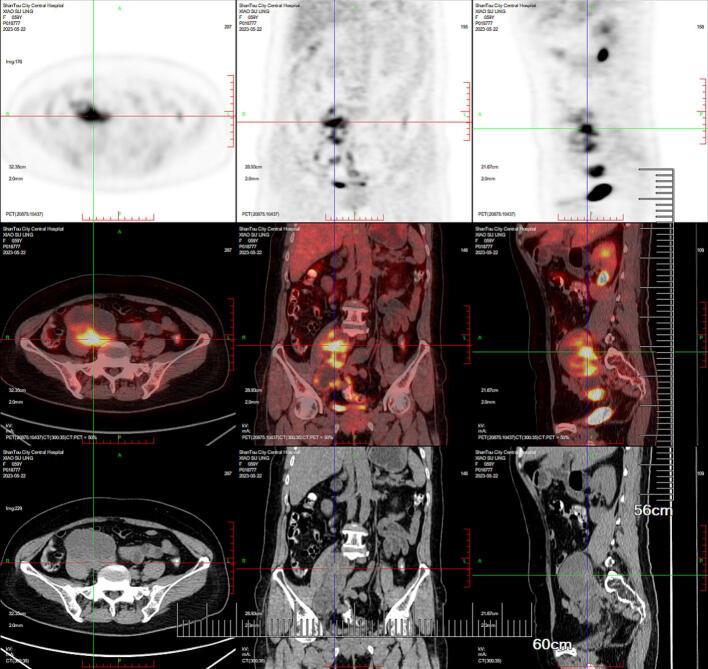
Positron emission tomography/computed tomography (PET/CT) shows a 7 × 6 cm heterogeneous mass in the right adnexal area.
